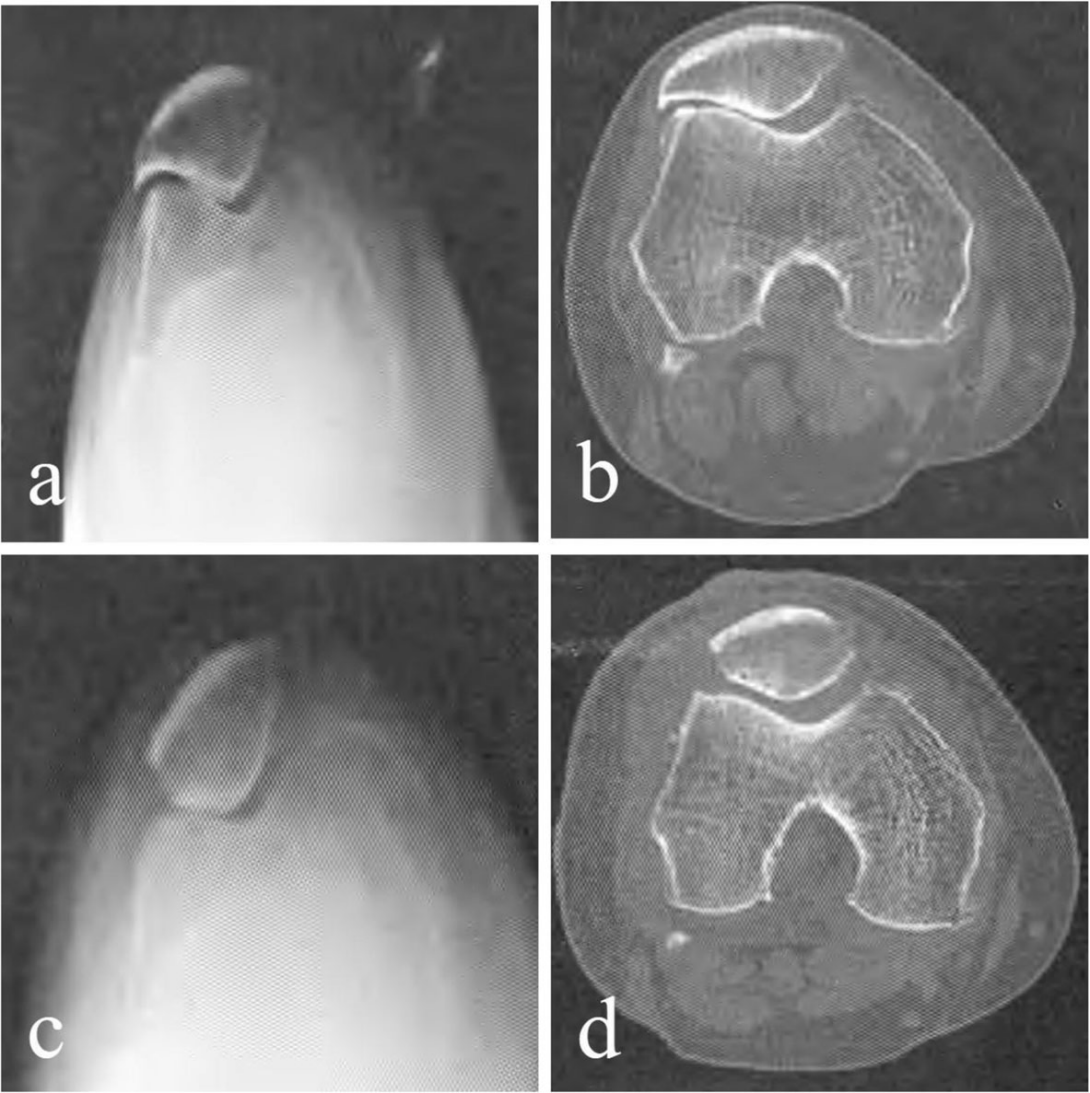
Comparison of preoperative and postoperative radiographic data of X-ray and CT examination of the ELPS patient. a, b Preoperative tangential X-ray imaging and CT tomography showed hook-shaped patella; the lateral space of the patellofemoral joint was significantly narrow and osteophyte formation was obvious. c, d Postoperative tangential X-ray imaging and CT tomography showed significant widening of the lateral space of the patellofemoral joint and its medial and lateral space restored to balance; the lateral hook-shaped patella had been shaped into a V-shaped patella; the osteophyte around the lateral patellofemoral joint had been removed and the joint returned to normal
